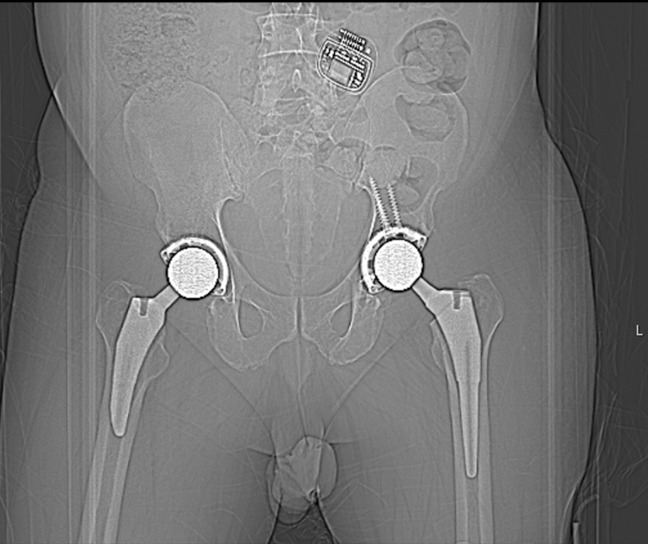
Postoperative radiograph of revision left total hip arthroplasty.
